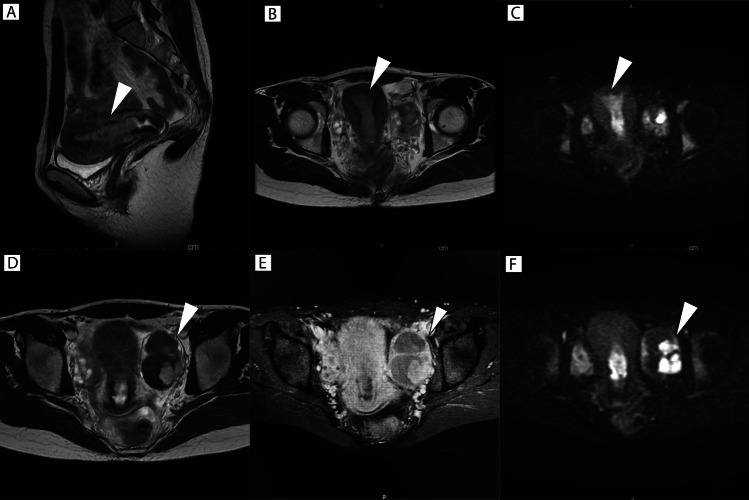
Pelvic magnetic resonance imaging findings at initial presentation. (A, B) Sagittal and axial T2-weighted images showing endometrial thickening (arrowheads). (C) Diffusion-weighted image showing restricted diffusion in the thickened endometrium (arrowhead), consistent with malignancy. (D–F) Axial T2-weighted, contrast-enhanced T1-weighted, and diffusion-weighted images, respectively, showing a left ovarian mass with cystic and solid components (arrowheads). The junctional zone is preserved, and no evidence of extrauterine or metastatic spread is observed
